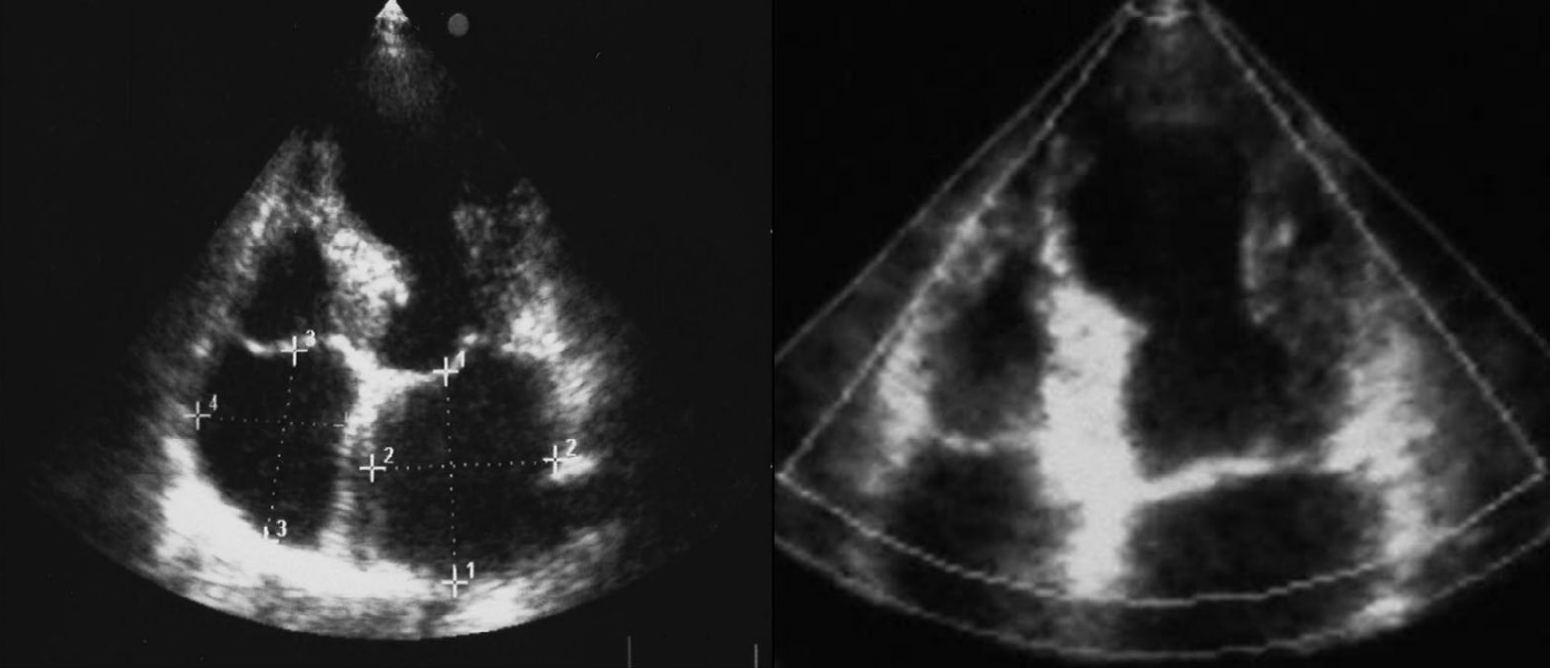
Transthoracic echocardiogram on admission. A. Apical 4-chamber view showing apical ballooning of the left ventricle. B. Zoom detail of the same view as in A.
